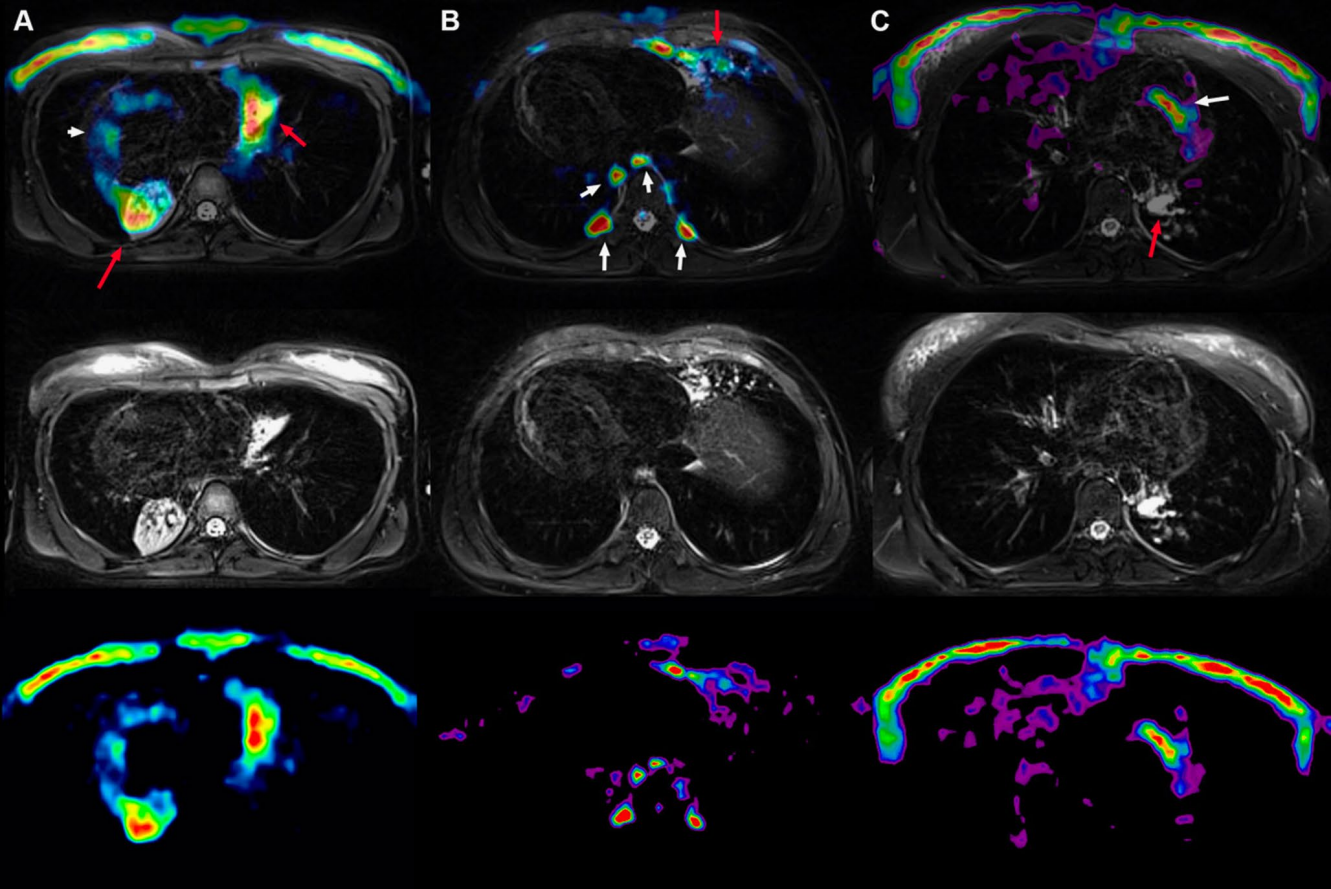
In the bottom row: parametric image (obtained from a PET not corrected for attenuation divided by the average count in a lung area not affected by the pathology). In the middle row: MR image (T2 blade fatsat respiratory triggered - TR:8830; TE:126; 1.18 × 1.18 mm). In the top row: fused image. In columns: three distinct patients: (A) female with dextrocardia showing high tracer metabolism in the para-cardiac areas (red arrows) corresponding to the alterations visible on MR and a non-specific cardiac uptake (white arrow); (B) male with dextrocardia showing intermediate uptake (red arrow) corresponding to the alterations visible on MR and a non-specific uptake corresponding to activation of paravertebral brown fat; (C) female showing absent metabolism corresponding to the areas of consolidation visible on MR (red arrow) and non-specific cardiac uptake (white arrow)
